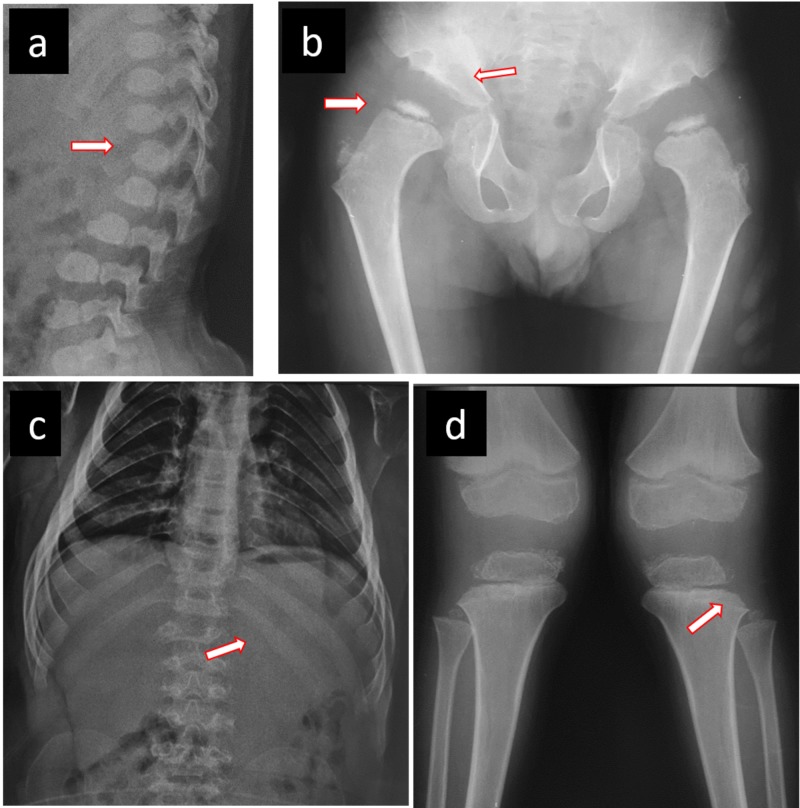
Radiographic features in an MPS IV A male patient a) Central beaking of the first lumbar vertebrae and posterior vertebral scalloping. b) Severe acetabular dysplasia, coxa plana, broad neck of femur. c) Flattened ribs, hepatosplenomegaly. d) Genu valgus with proximal and lateral tibial dysplasia, widening of the physeal line. MPS: mucopolysaccharidosis
